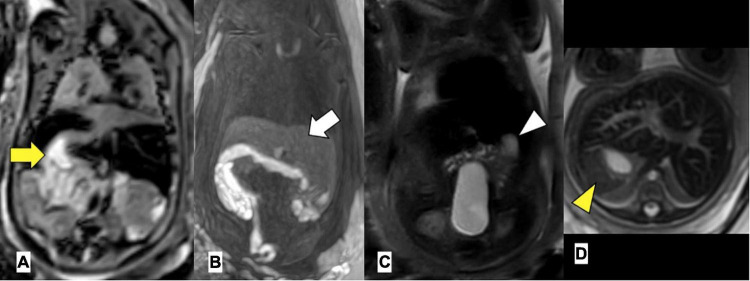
Left atrial isomerism by foetal MRI. (A) Arrow yellow: right-sided stomach (B) arrow white: bilateral liver, (C) arrowhead white: left-sided gallbladder, (D) arrowhead yellow: left-sided spleen. Gestational age at MRI is 20 weeks + 0 day.
